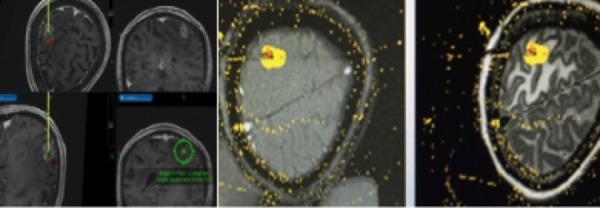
Stealth Plan Stealth plan for insertion of the Visualase laser into the lesion (left). The final ablation result is seen on the T1 and fluid-attenuated inversion recovery (FLAIR) axial MRI scans, which demonstrate minimal thermal damage to surrounding tissue (right).
